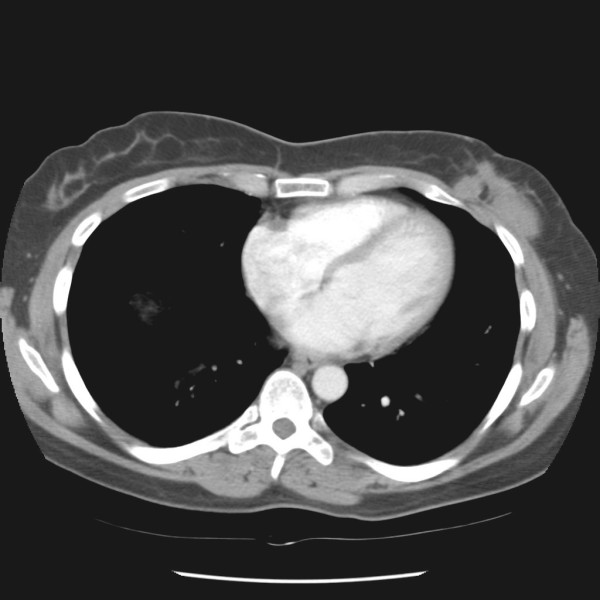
Computed tomography scan axial view.
